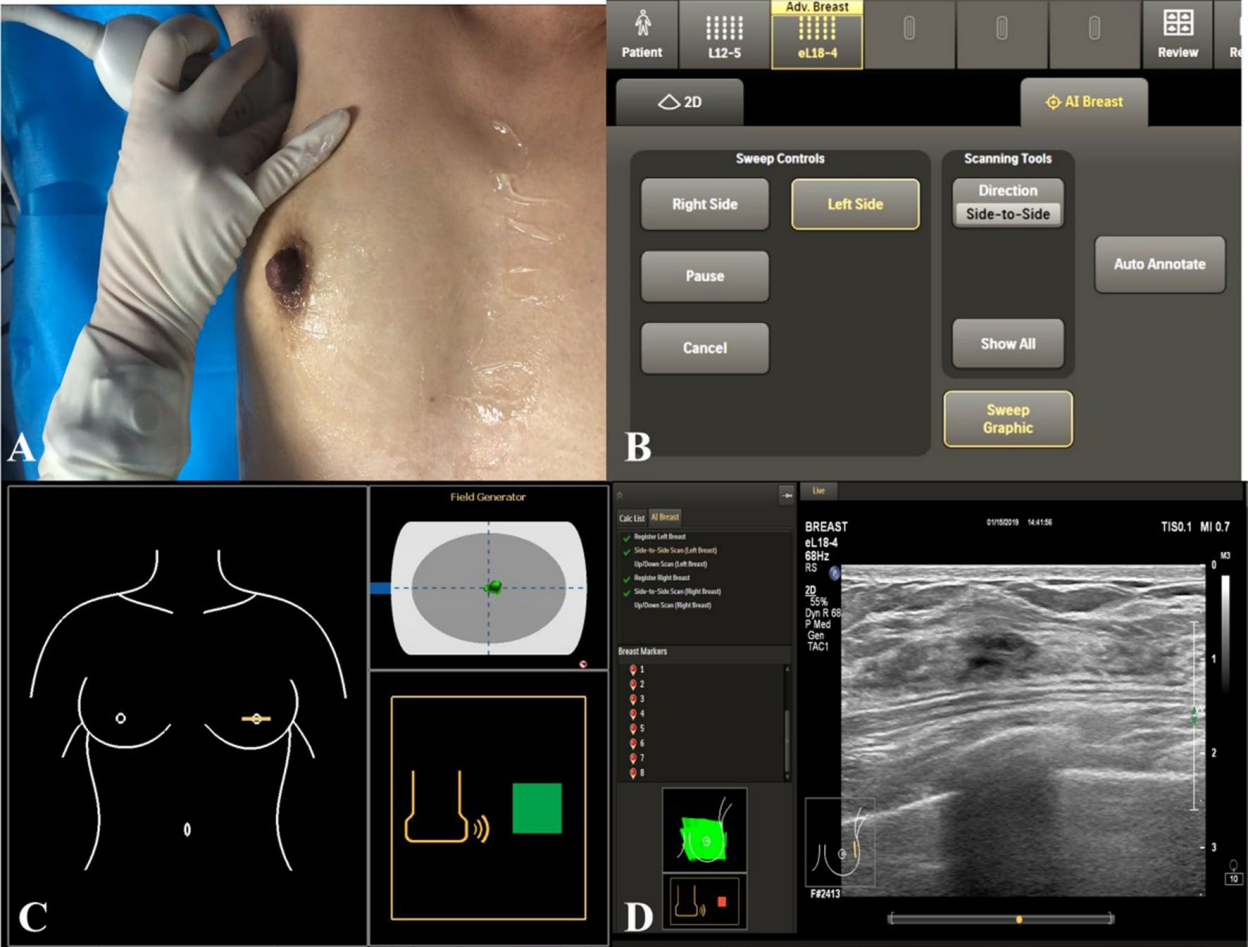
AI breast ultrasound scan method. A -patient maintained a supine position; B- and C- transducer marker verification on the on-screen breast graphic; D- image acquisition
